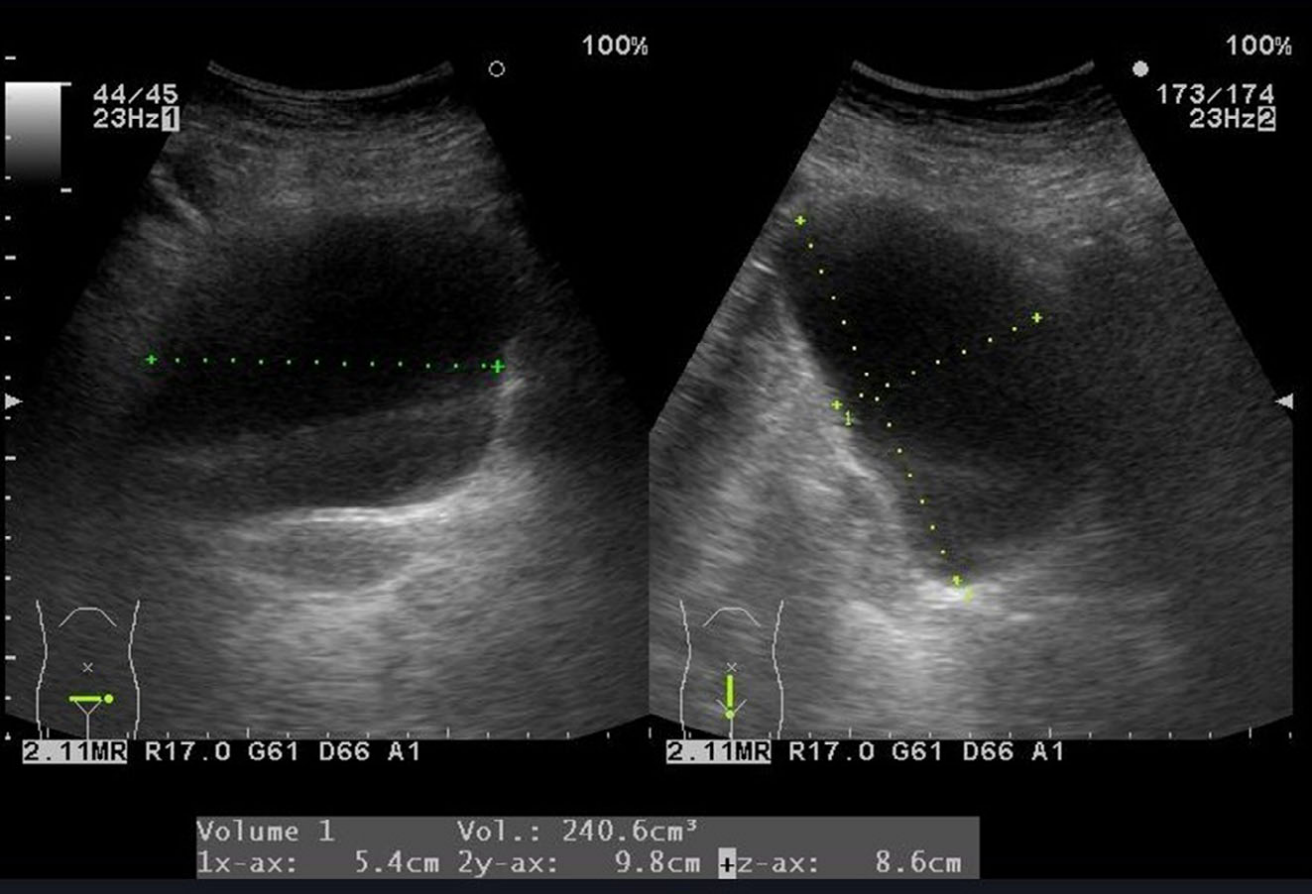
Abdominal ultrasonography showing a hypoechoic area in the right suprapubic area and a postvoid residual urine volume of 240 mL. [Colour figure can be viewed at wileyonlinelibrary.com]
